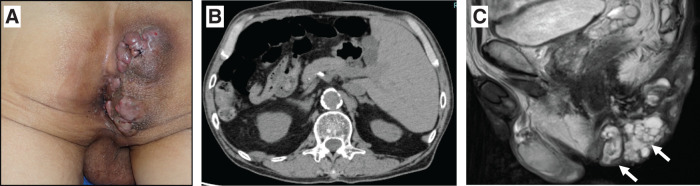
Preoperative findings. (A) Perianal region with cutaneous lesions. (B) CT revealed situs inversus totalis. (C) Primary region on MRI (arrow).
